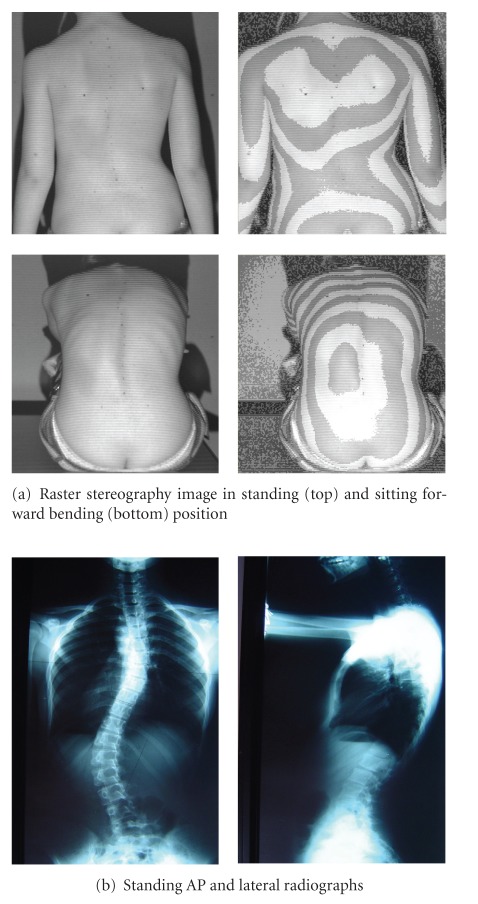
13 years and 9 months old girl, thoracic Cobb angle 26°, thoracolumbar Cobb angle 46°, Risser sign 4. 1.5 years after menarche. Main curve ATR = 15°, sum of three ATRs = 20°, POTSI = 50.2, HS = 18, (a) raster stereography, (b) radiographs.
